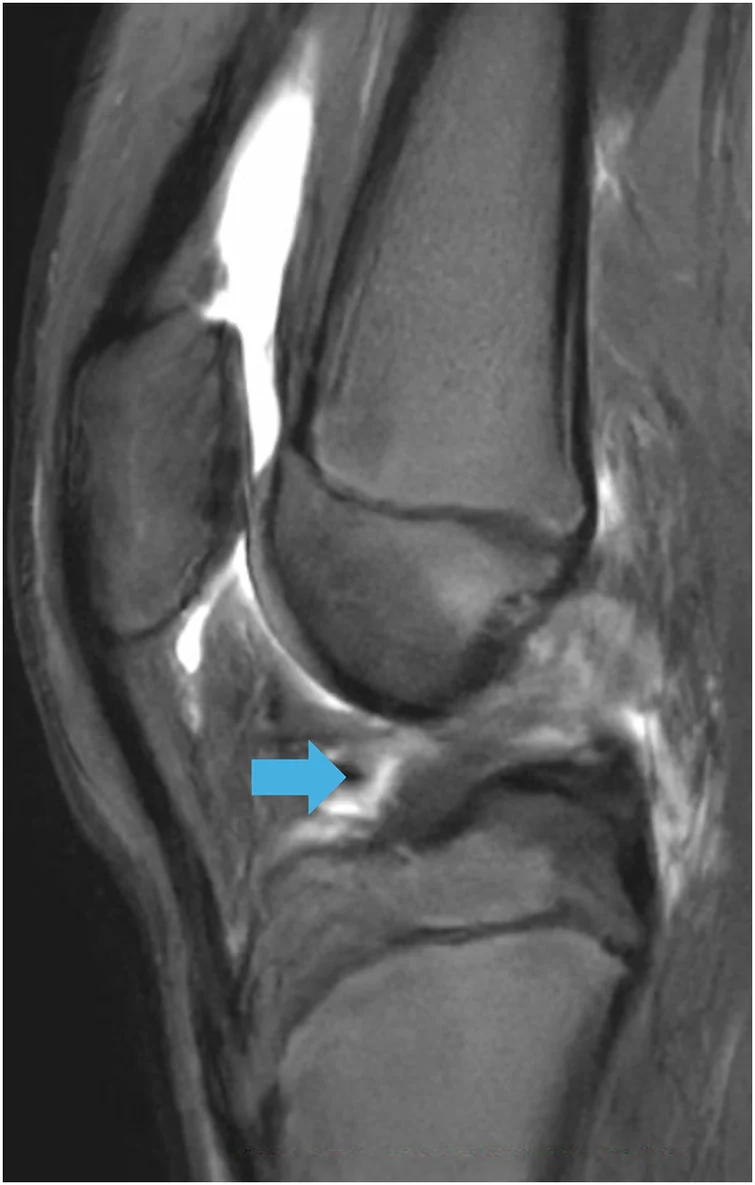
MRI findings associated with ACL tear on a T2 sagittal image of the knee. The ACL (arrow) shows abnormal takeoff with fibers that diverge from the Blumensaat line. In addition, increased signal intensity and discontinuity of the fibers can be seen at the femoral insertion.
